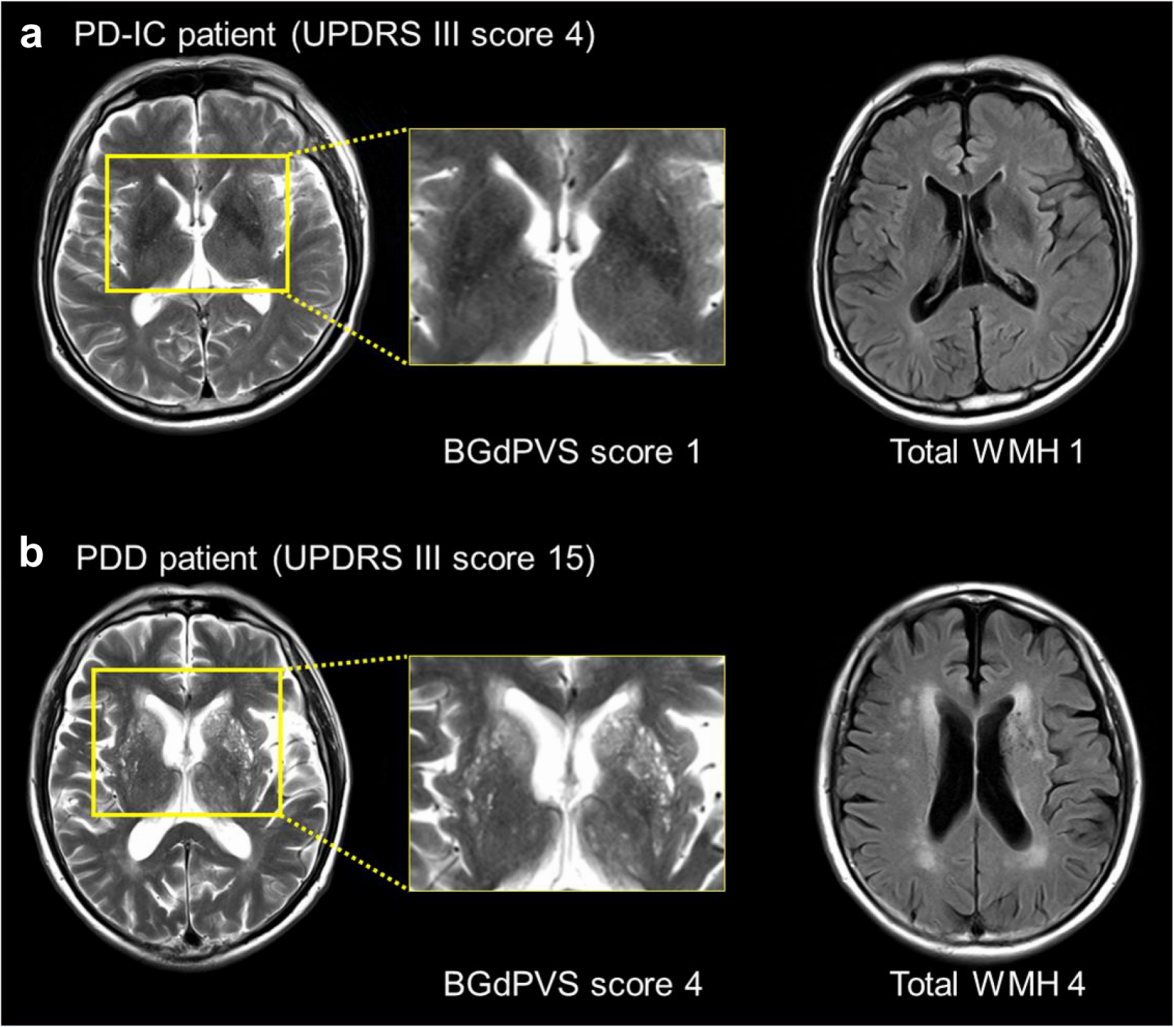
Representative cases showing the associations of BGdPVS and WMH with cognitive status and motor symptoms. a A 72-year-old male diagnosed as PD-IC, with a UPDRS III score of 4. The patient did not have a history of hypertension or SH. The axial T2-weighted images show a BGdPVS score of 1. On FLAIR images, the total Fazekas score of WMH was 1. b A 79-year-old male diagnosed with PDD, with a UPDRS III score of 15. The patient had hypertension and SH. The axial T2-weighted images show BGdPVS score of 4. On FLAIR images, the total Fazekas score of WMH was 4. Abbreviations: BG basal ganglia, dPVS dilated perivascular spaces, FLAIR fluid-attenuated inversion recovery, PD-IC PD with intact cognition, PDD PD with dementia, SH supine hypertension, UPDRS III Unified Parkinson’s Disease Rating Scale Part III, WMH white-matter hyperintensities.
